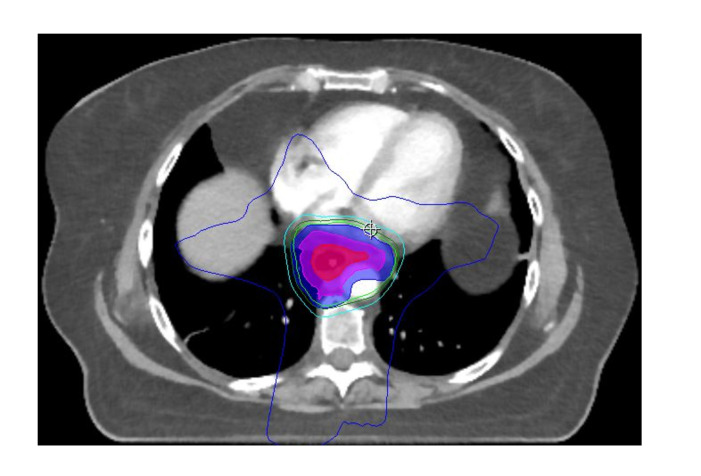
CT-based radiotherapy planning image of a distal oesophageal tumour
